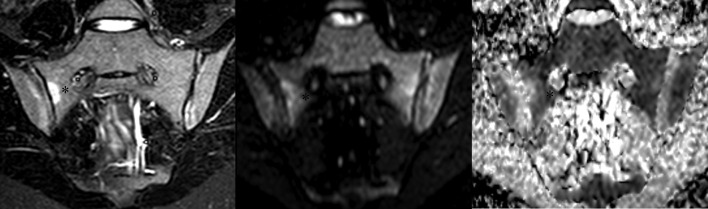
Oblique axial STIR (left), DWI (middle) and ADC (right) of the sacroiliac joints. A bone marrow edema is present in the sacral part of the right SI joint (asterisk). There is a similar finding on DWI (*b* = 50 s/mm^2^), whereas the lesion is larger on the ADC map. In the iliac part of the right SI joint a thin subchondral BME is present on STIR and DWI, while larger on the ADC map. ADC, apparent diffusion coefficient; DWI, diffusion-weighted imaging; SI, sacroiliac; STIR, short-tau inversion recovery.
